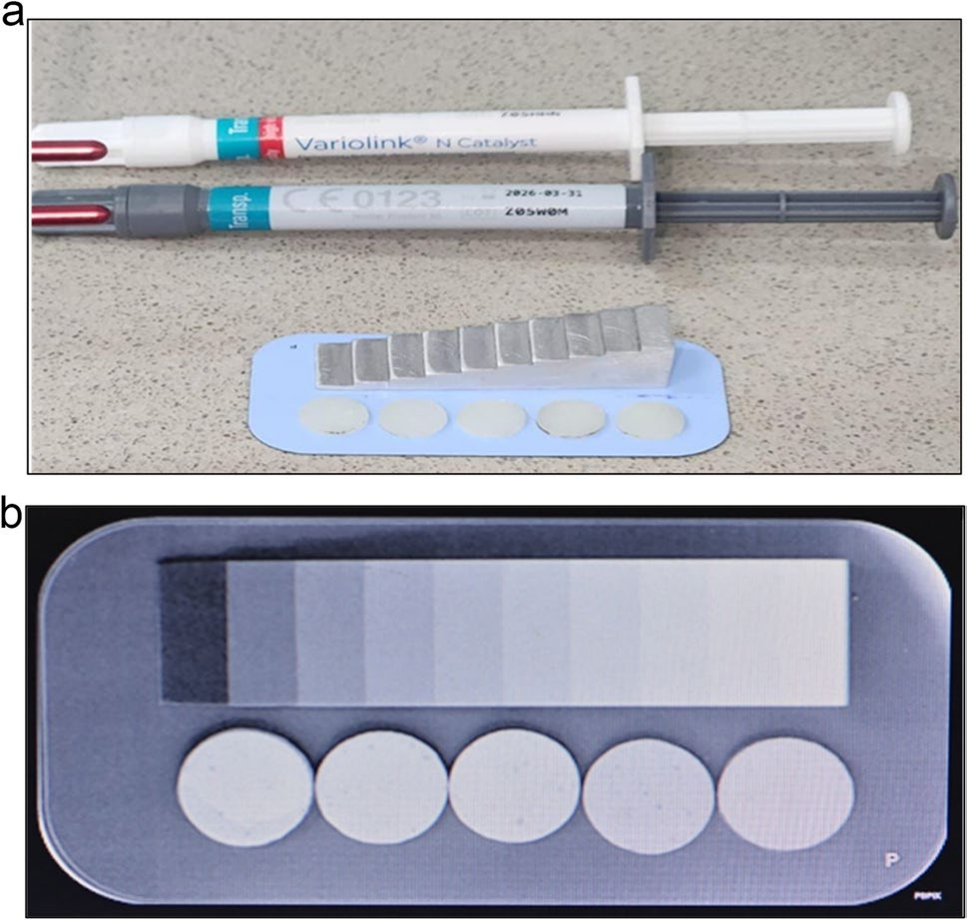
a Representative discs of dual-cure resin cement (NX3 Nexus^®^ and Variolink N^®^) positioned on the digital imaging plate for radiographic acquisition b Ten-step aluminum wedge (1–10 mm) used as the radiopacity reference standard
